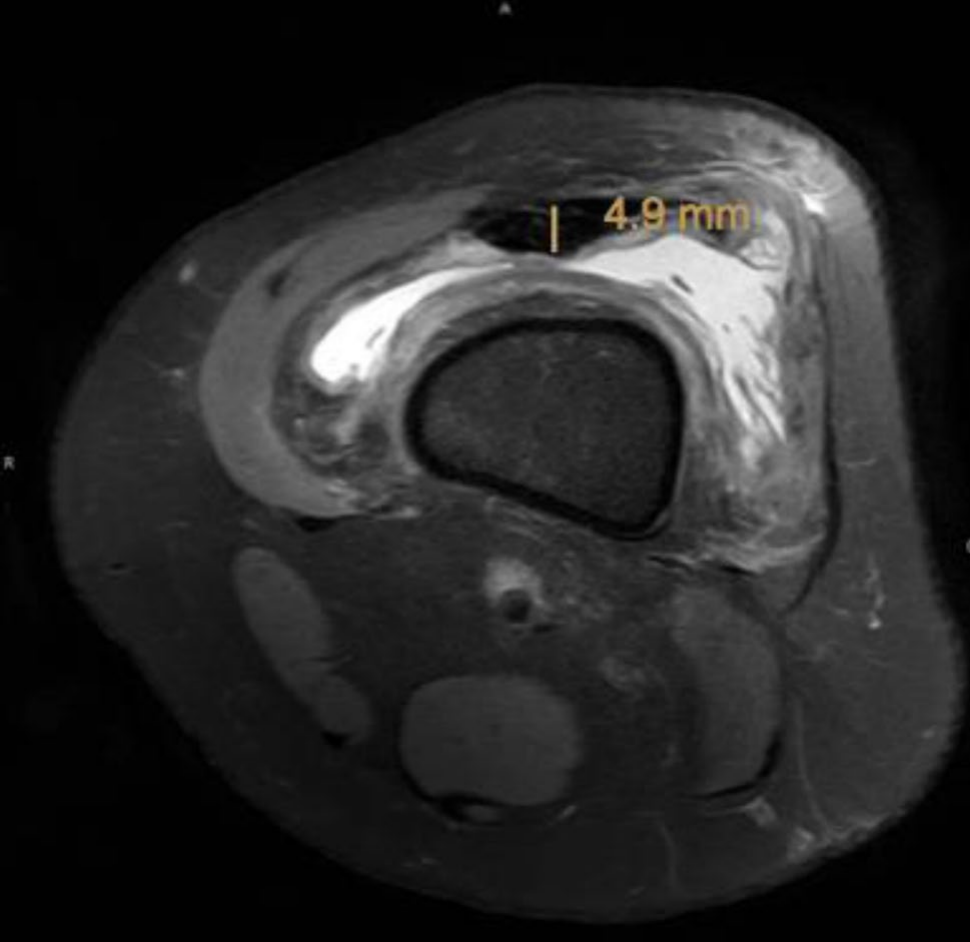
Thickness of the quadriceps tendon (QT) on an axial MRI (T2 sequence) measured 2 cm above the insertion of the tendon at the cranial patella pole
